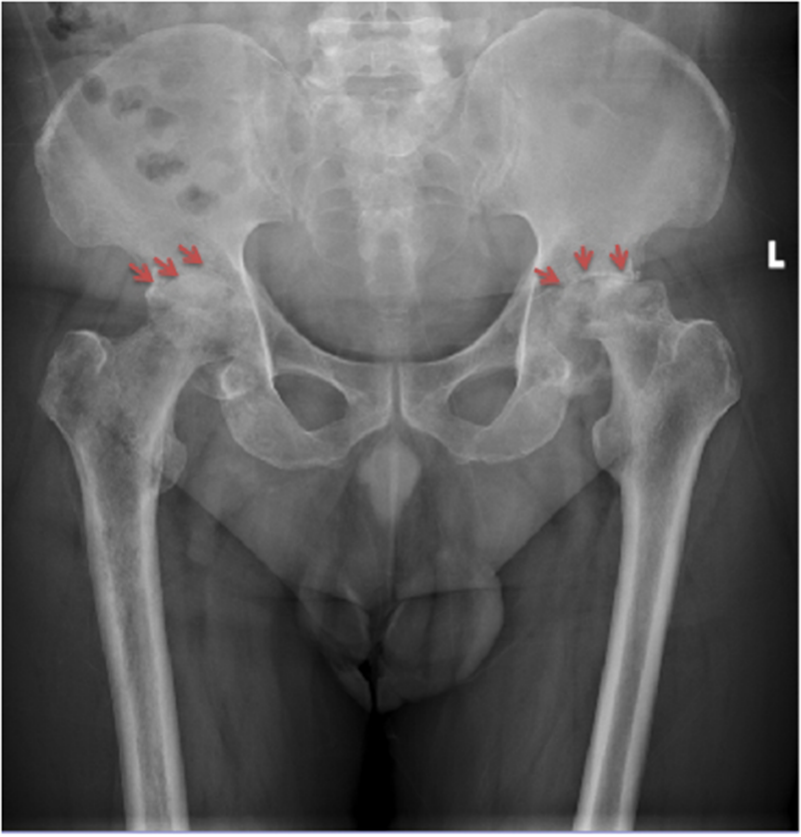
Patient 5, a 52-year-old male, anteroposterior radiograph of the hip revealed severe joint space narrowing, disappearance of the acetabular sourcil, and bone destruction in the femoral head and femoral neck. Images were taken six months after the onset of hip pain and fever.
